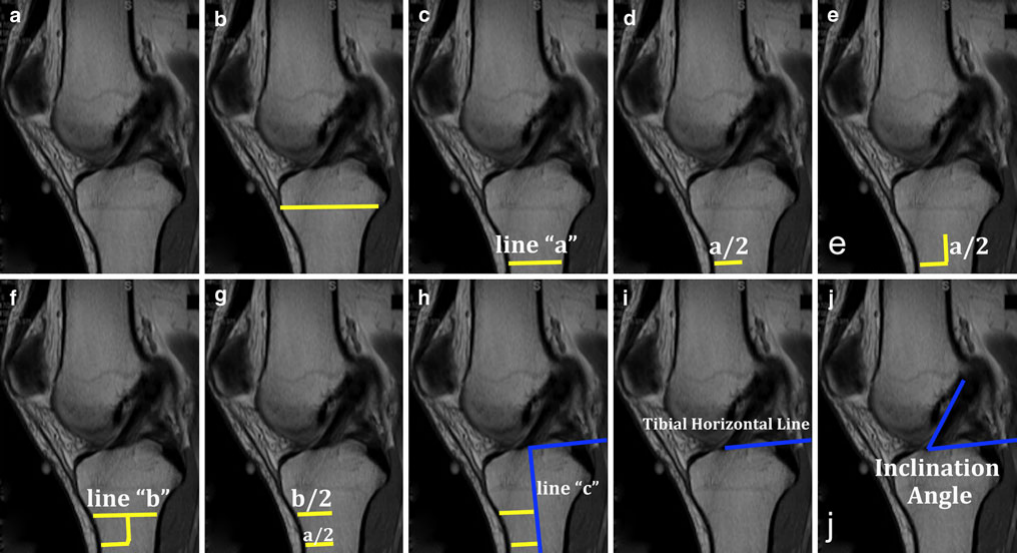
a Sagittal MRI showing the ACL and physeal scar; b line drawn over the physeal scar; c
